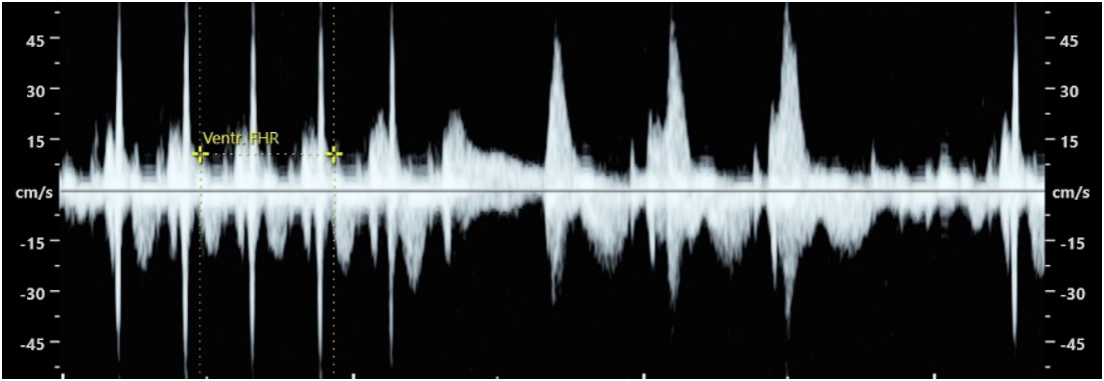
Fetal echocardiogram (Flow Doppler) at 21 weeks of gestation, revealing fetal supraventricular tachycardia alternating with sinus rhythm (a more explicit video is presented in Supplementary Material).
